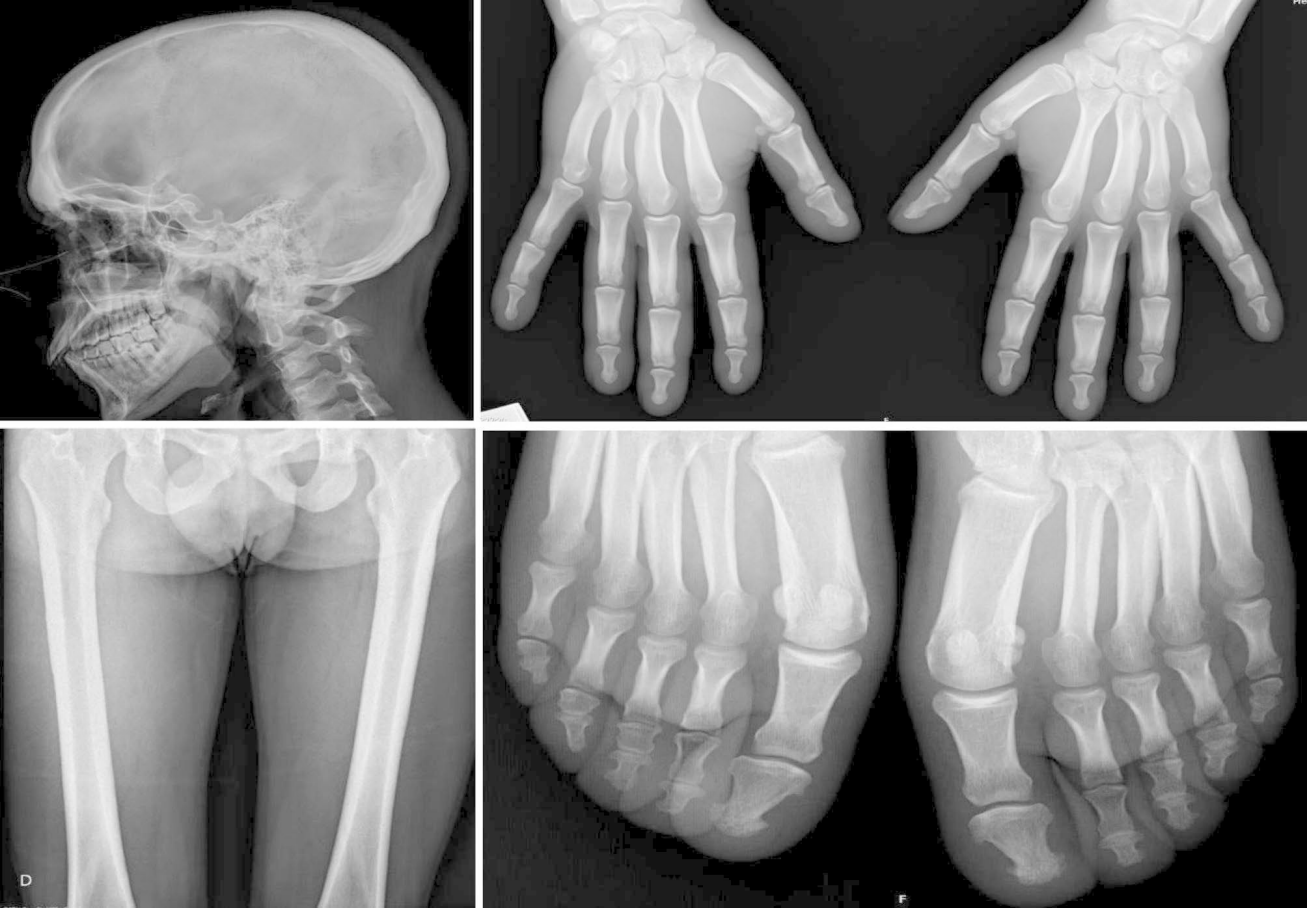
Plain radiographs show marked soft tissue swelling and periosteal hypertrophy with subperiosteal bone formation of the skull, femur and phalanges
